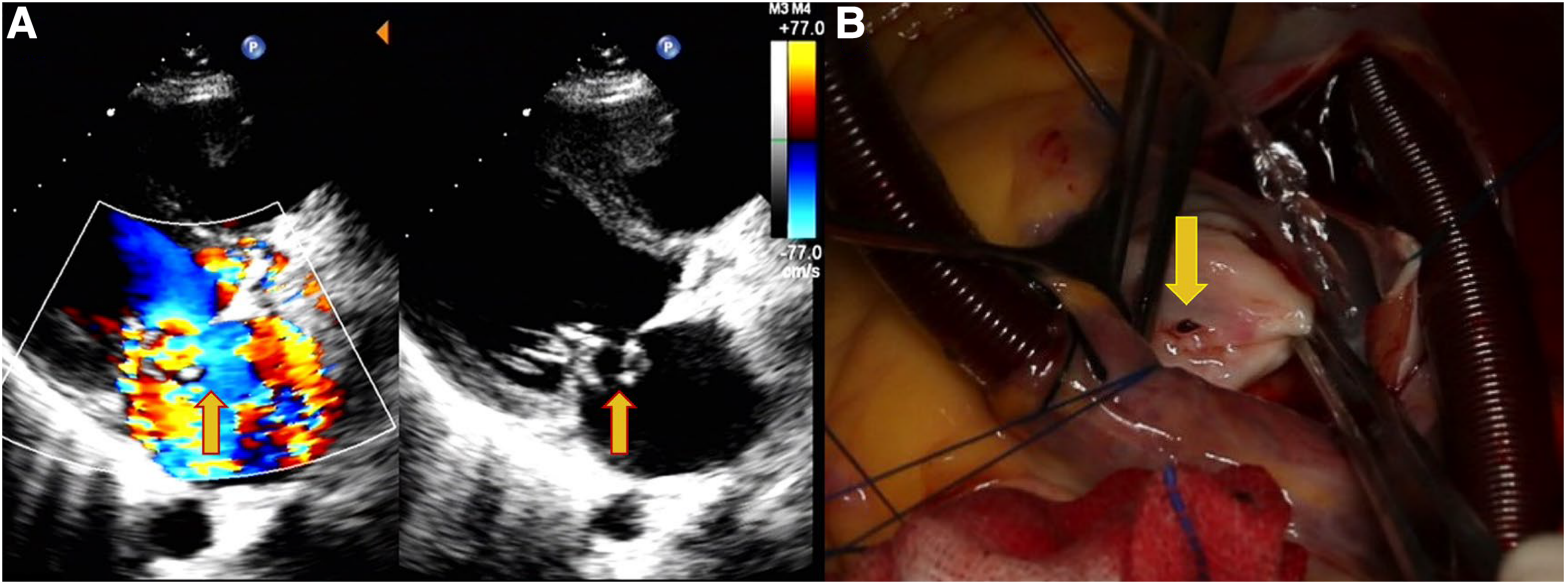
Echocardiography data of a 31-year-old female patient with shortness of breath, palpitations, and fever for 7 days. (A) Perforation of the MVA and regurgitation (yellow arrows). (B) Site of the MVA perforation during surgery (yellow arrows).
